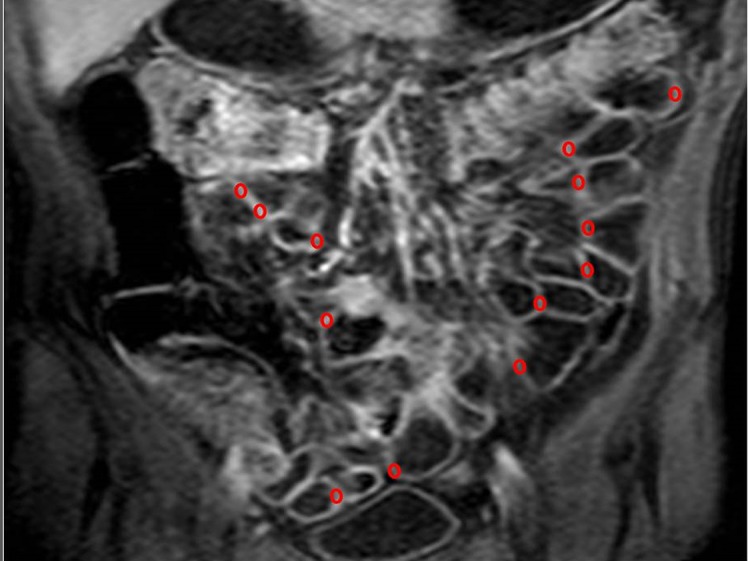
Assessment of small bowel wall perfusion. To calculate MRI perfusion parameters, AUC_60_, AUC_90_ and K^trans^, elliptic ROIs were positioned in different segments of the small bowel wall, particularly on proximal and distal jejunum and proximal, middle and distal ileum, as showed in this figure.
